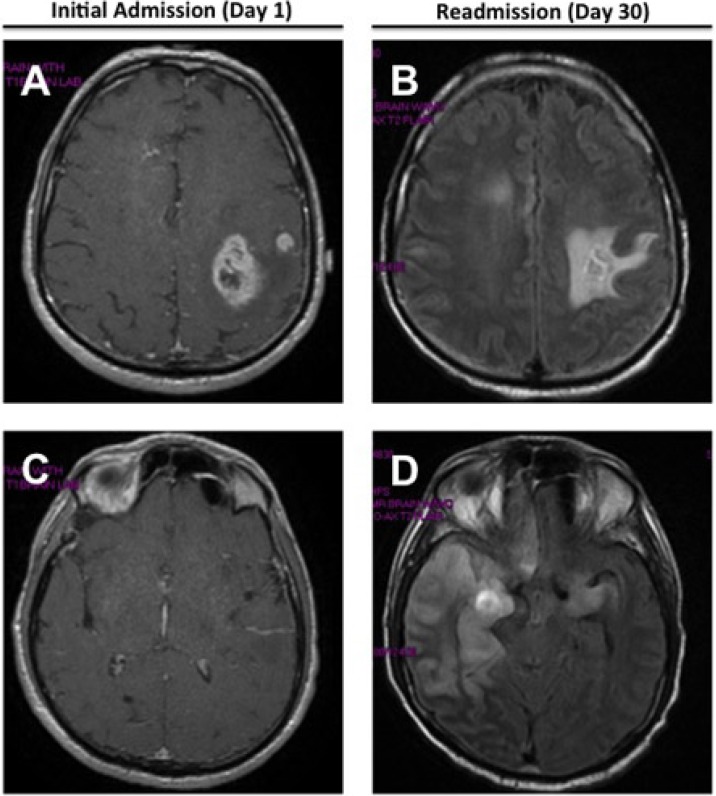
Axial MR images of brain with contrast demonstrating T1 weighted left temporoparietal enhancing lesions on initial presentation (A,C), and T2 FLAIR right frontotemporal signal abnormalities on hospital readmission (B,D).
